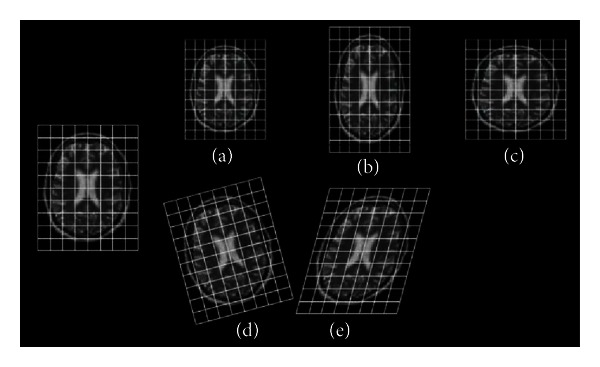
Left image: original MRI brain transaxial image. (a) Balanced diagonal elements result in global rescaling. (b) Unbalanced diagonal elements produce anisotropic rescaling along the *y*-axis. (c) Unbalanced diagonal elements produce anisotropic rescaling along the *x*-axis. (d) Specific balanced relations between off-diagonal elements in the matrix produce rotations. (e) An unbalance change in the off-diagonal elements resulting in shears along one of the two axes.
